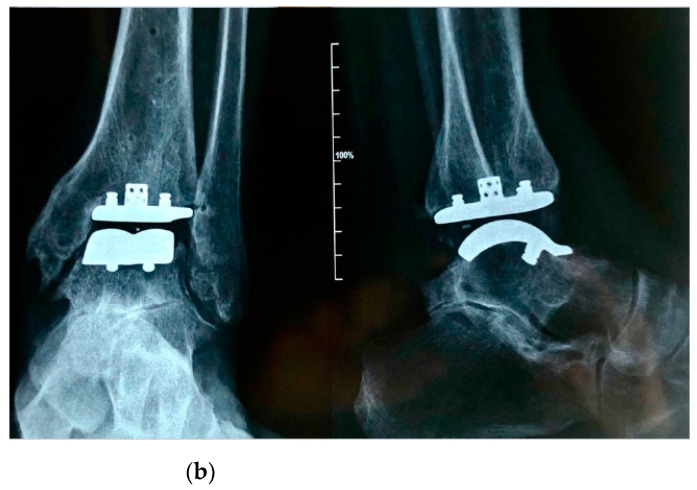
Pre-operative radiographic image in anteroposterior and lateral view (on the left and on the right, respectively) of an arthritic ankle joint, with a slight articular varus deformity (a) and post-operative radiographic results (b).
